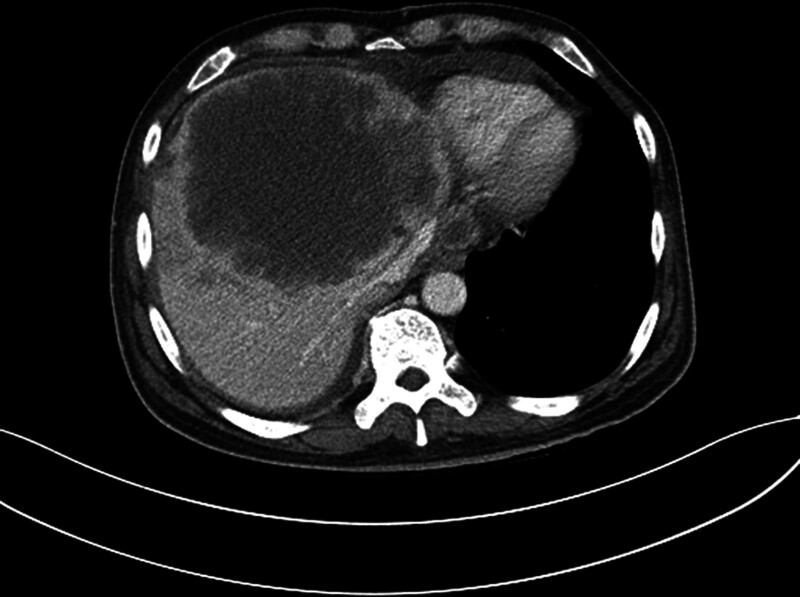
Contrast-enhanced abdominal CT revealing ill-defined heterogenous enhancing hepatic mass more than 12 cm × 11 cm.
